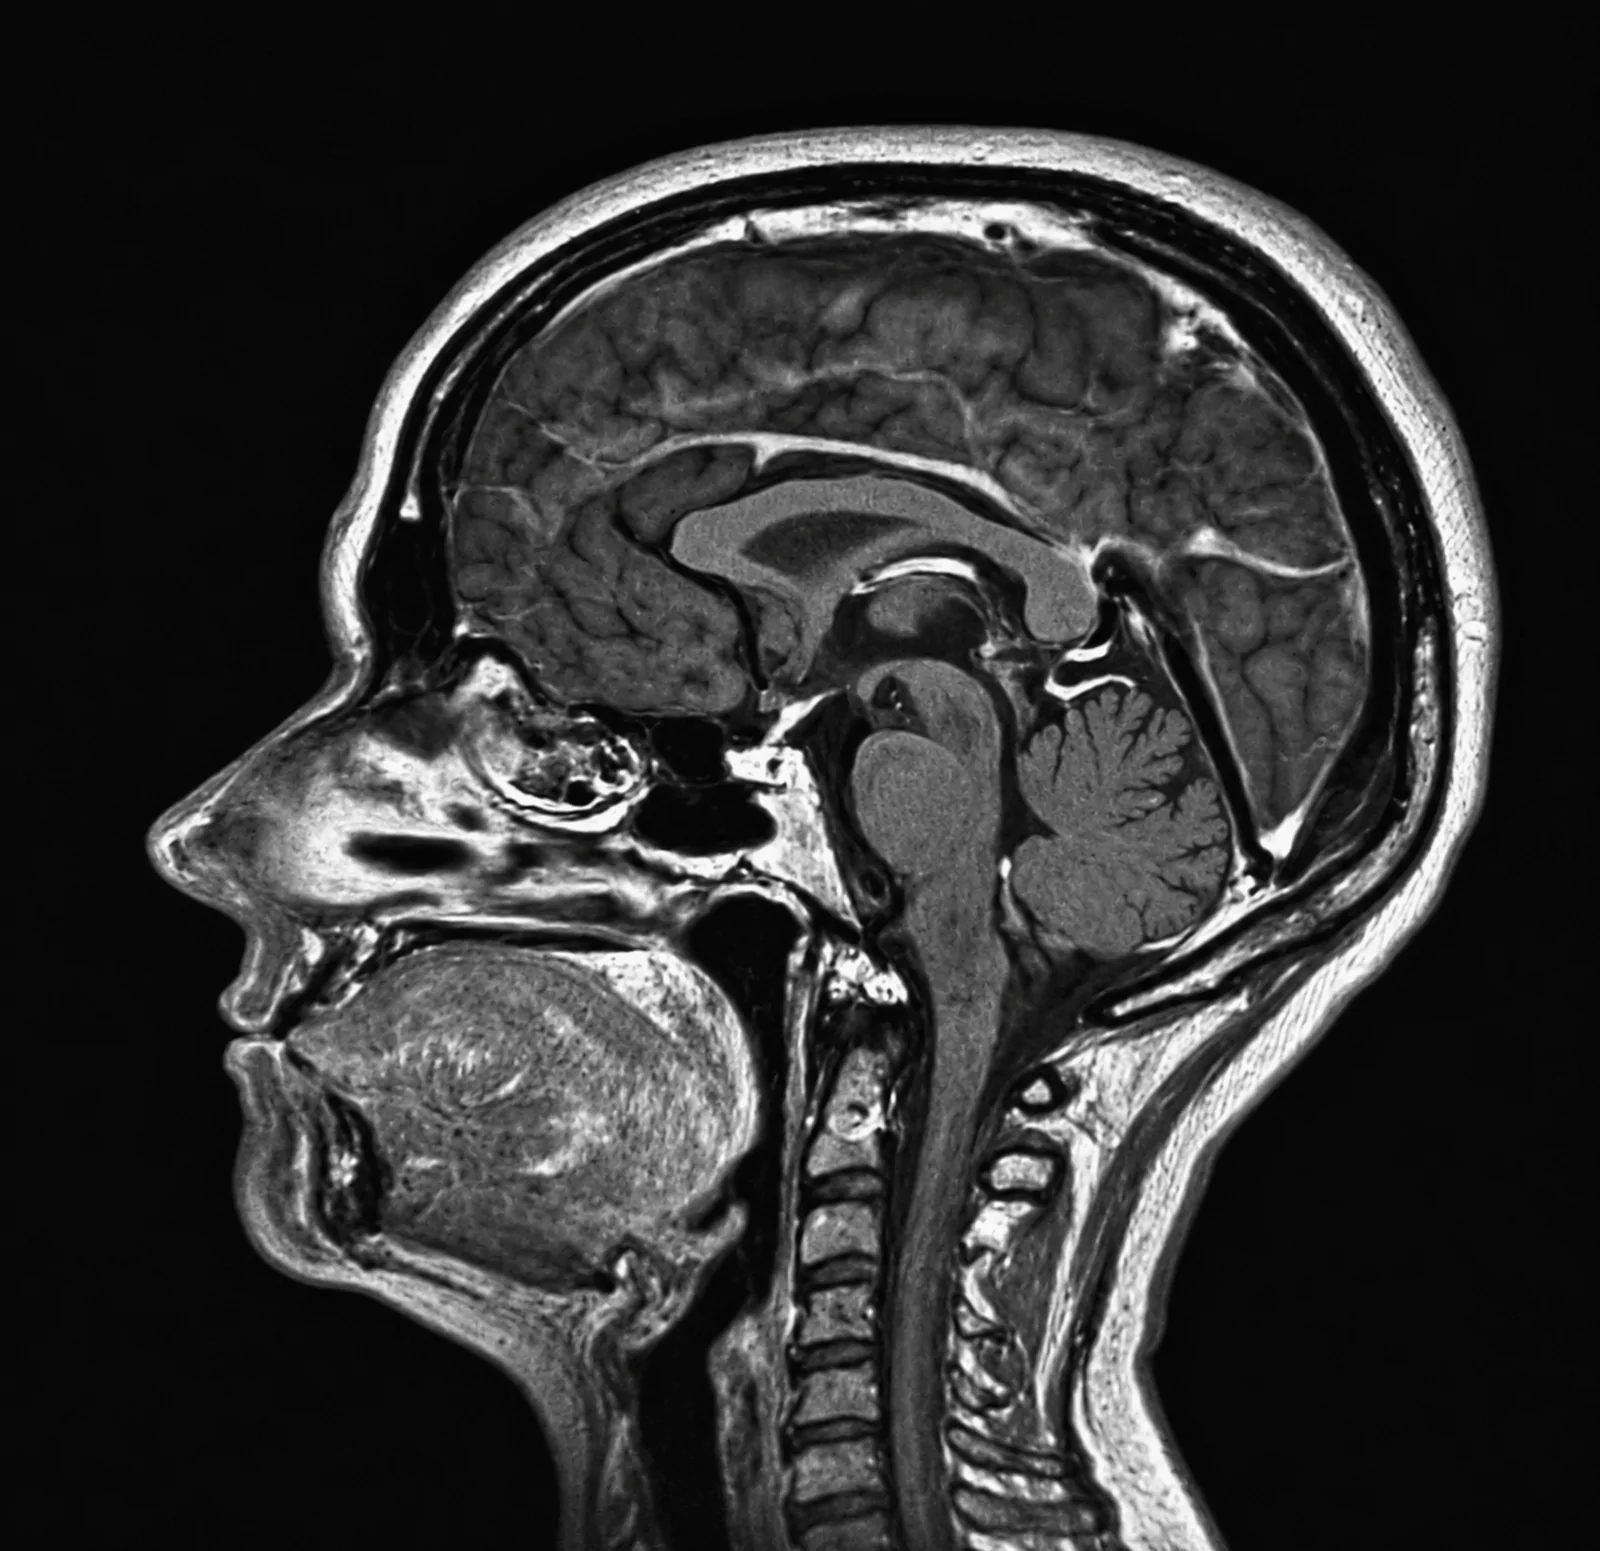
Magnetic resonance imaging of the head, sagittal view, which did not demonstrate intracranial pathology
